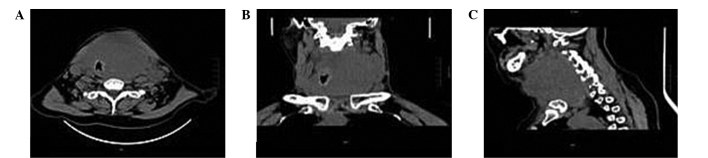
Multi-planar reconstruction computed tomography image of the neck shows a significantly enlarged thyroid, particularly in the left lobe and the isthmus, with reduced tissue density. The lesion extends from the upper level of the third cervical vertebra to the arterial arch, and presses the surrounding structures toward the right side. (A) Transversal view, (B) coronal view and (C) sagittal view.
